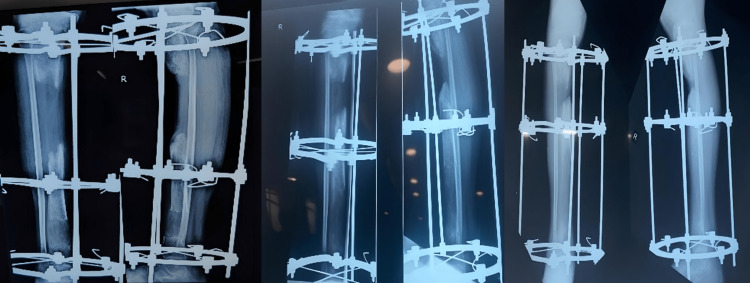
X-rays when the patient came for regular follow-ups subsequently every month.
